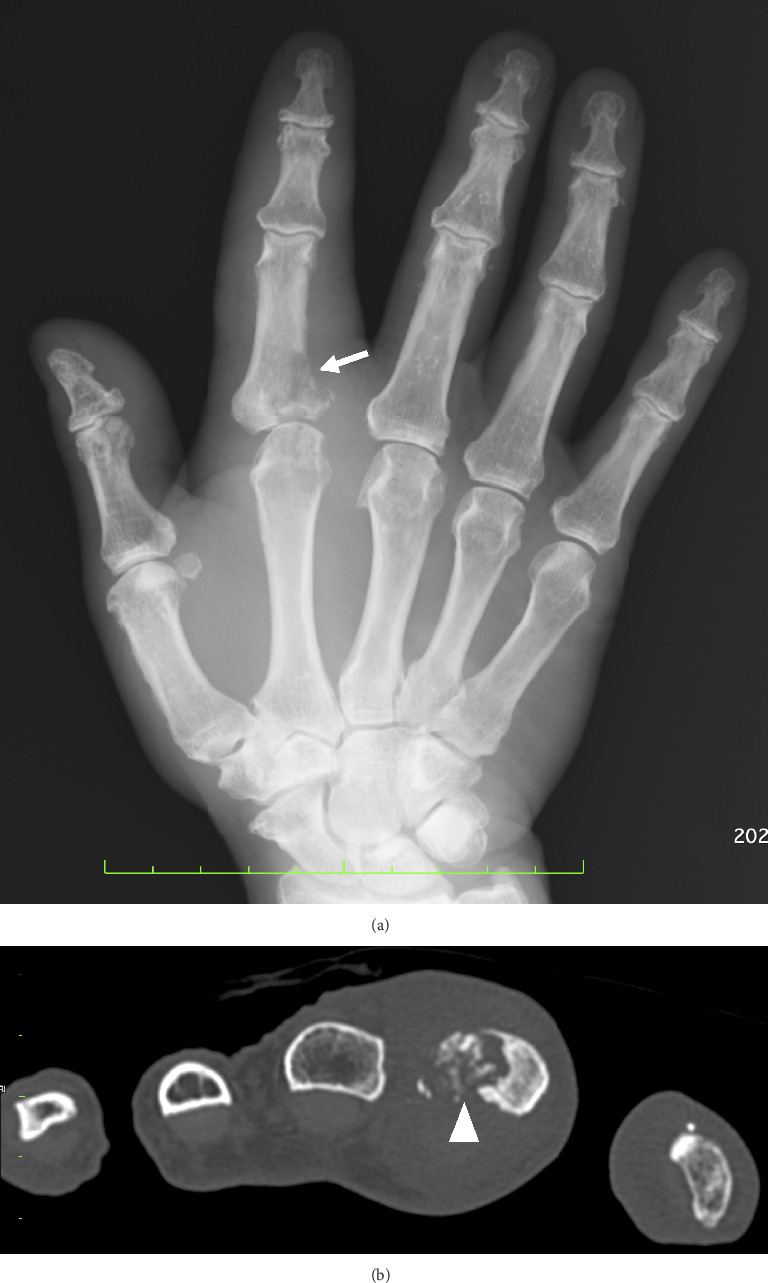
(a) Roentgenogram of the right hand. Bony destruction of the ulnar aspect of the base of the proximal phalanx of the right index finger was observed (arrow). (b) Computed tomography of the right hand. Bone destruction of the ulnar aspect of the base of the proximal phalanx of the right index finger was observed (arrowhead).
